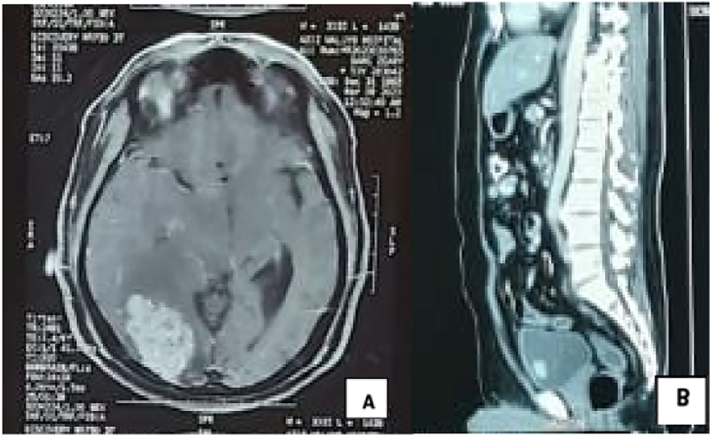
(A) Computed Tomography Scan of the brain showing meningioma, and (B) bladder mass found in CT Scan Imaging.
